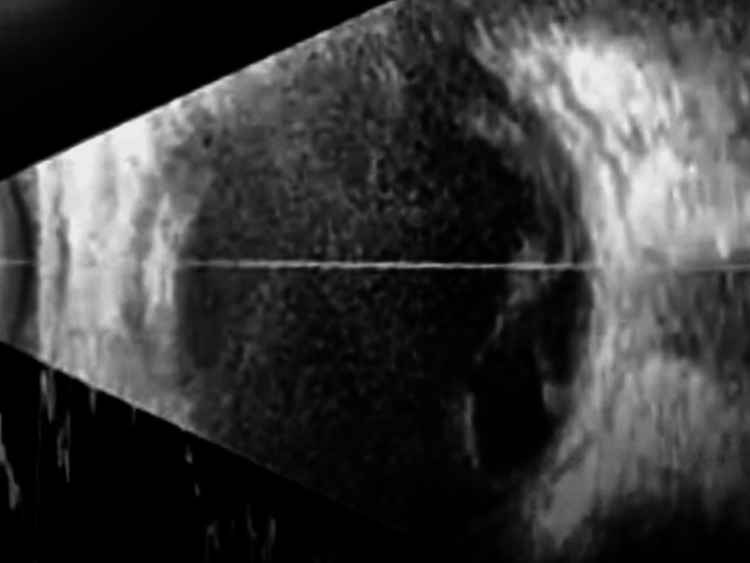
Left eye B-scan ultrasonography showing dense vitritis with loculation and fluid in the sub-Tenon space.
